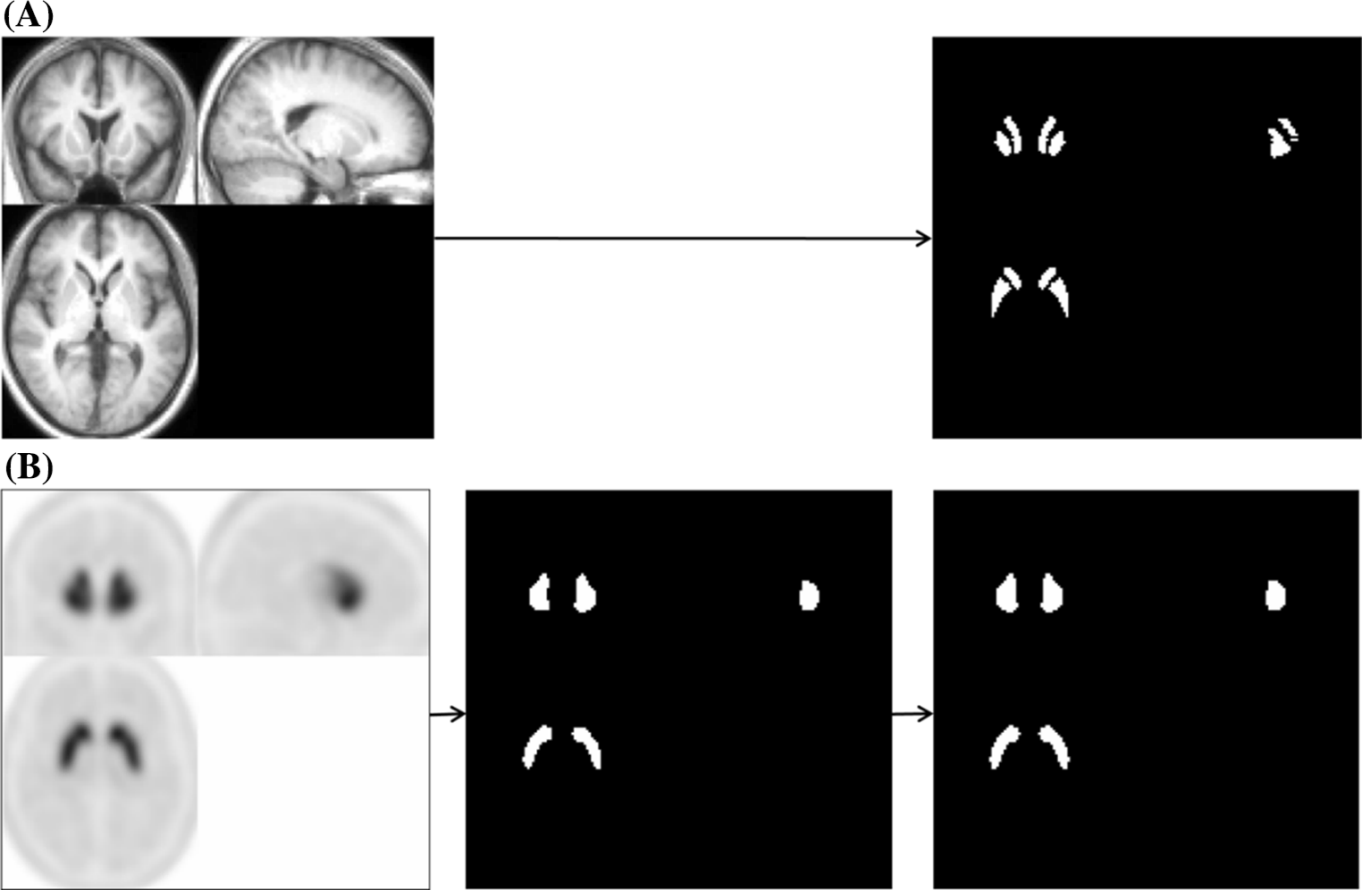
Methods of creating MRI-based ROIs (*upper row*: a) and I-123 FP-CIT-based ROIs (*lower row*: b). a MRI-based ROIs were set manually on the right striatum, and then mirrored. b I-123 FP-CIT SPECT-based ROIs were created via the automatic extraction of the striatal region at various cut-off levels. The ROIs were then fused with the reversed ROIs to ensure symmetry
